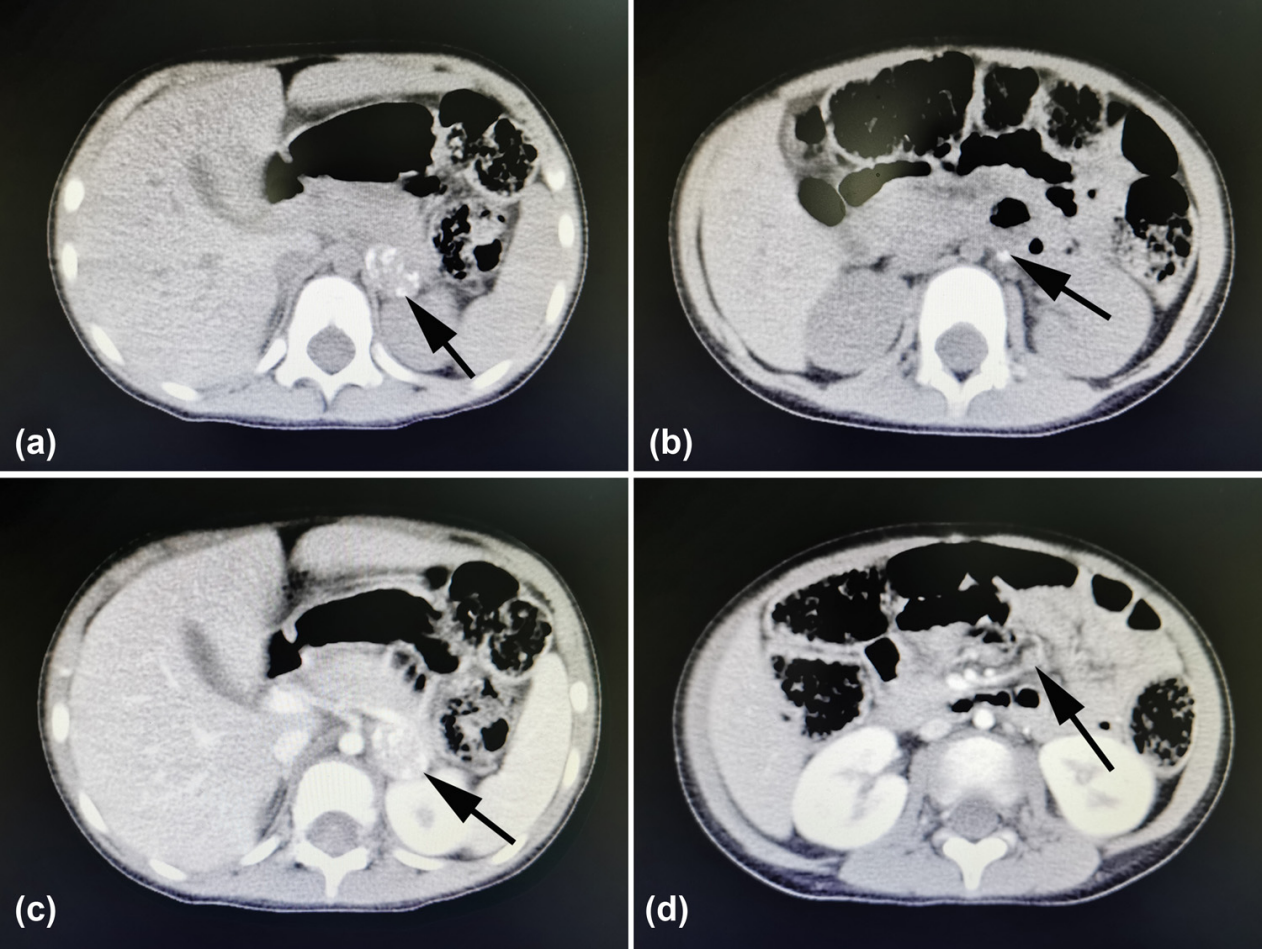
Abdominal CT findings. (a) A plain scan showing a left adrenal tumor of approximately 1.5 cm × 2.2 cm × 2.7 cm size (black arrow); (b) a plain scan showing a calcified nodule on the left side of the abdominal aorta (black arrow); (c) an enhanced scan revealing left adrenal tumor with increased calcification inside (black arrow); (d) an enhanced scan showing the mesenteric lymph nodes (black arrow).
